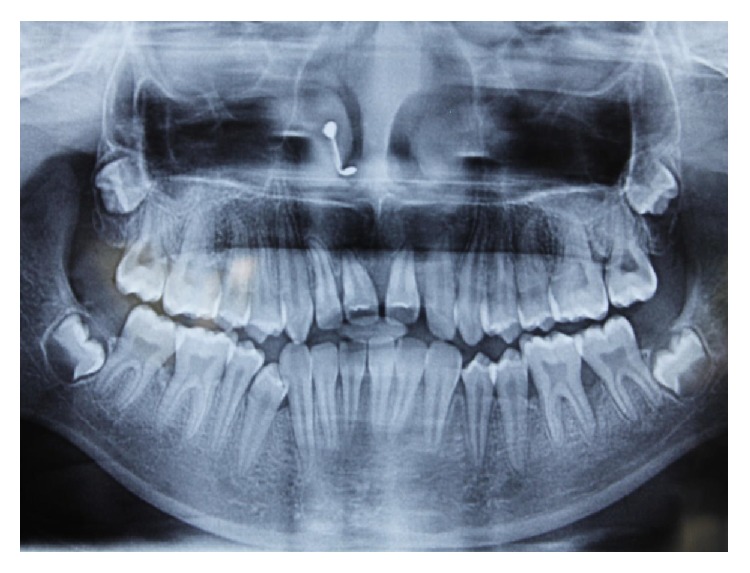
Orthopantomograph of the patient.
